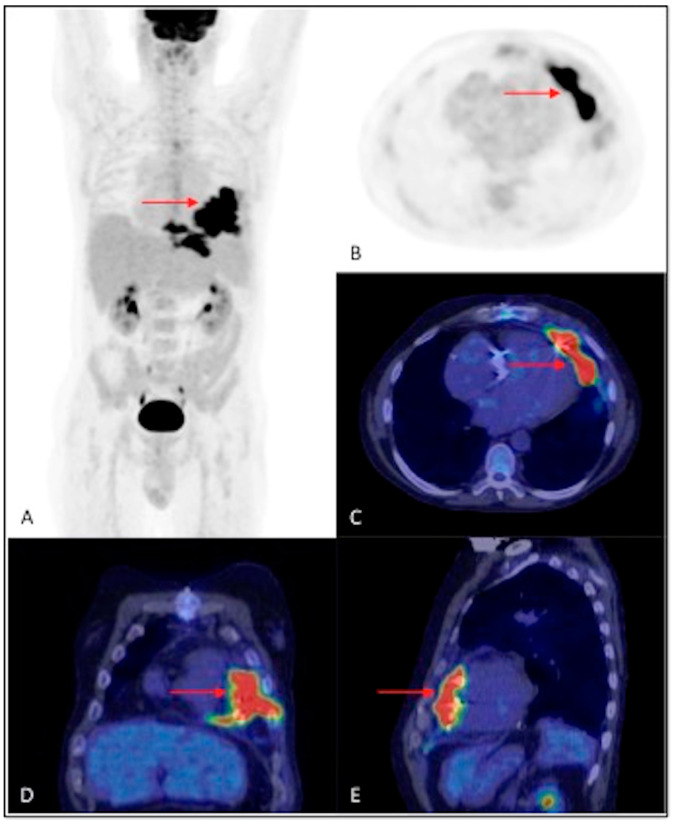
^18^F-FDG PET/CT of a 48-year-old man with implanted PM, with suspected CIEDI for therapy-resistant hyperpyrexia and positive blood culture for *Pseudomonas aeruginosa*. (A) MIP, (B) axial PET, (C) axial fusion, (D) coronal fusion, and (E) sagittal fusion images demonstrate the presence of intense hyperaccumulation of ^18^F-FDG along the pericardial part of the lead (SUV_max_ 25.5) (red arrows). The patient underwent extraction of the device and microbiological analysis confirmed the bacterial infection.
